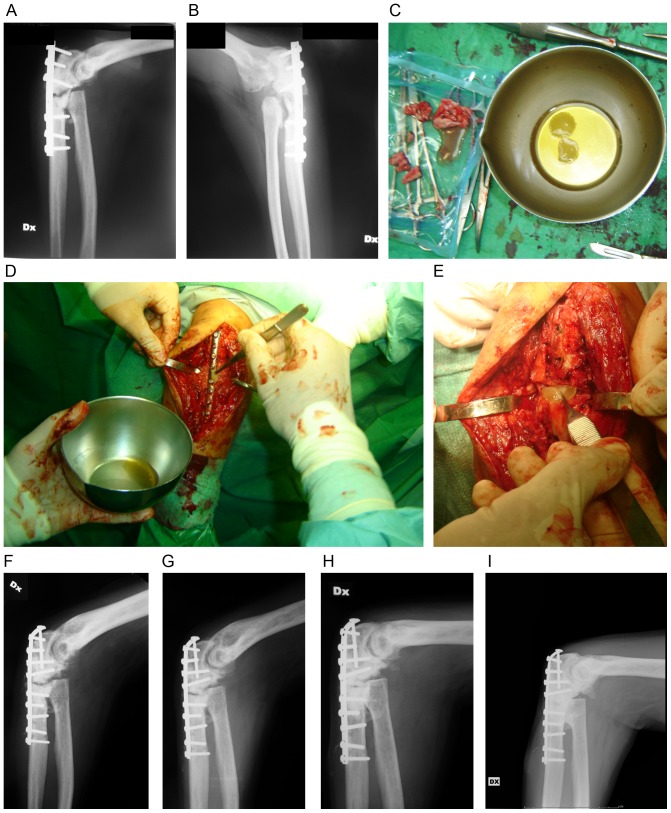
Pre- and post-implant of MSC/fibrin clot constructs in a representative patient (M.51). (A,B) Pre-intervention images showing pseudarthrosis and plate detachment. (C) MSC/fibrin clot constructs and bone chips on the operating table. (D,E) Implant of MSC/fibrin clot constructs during surgical procedure. Radiographic follow-ups at: (F) 2 months; (G) 6 months; (H) 9 months, showing occurred healing and (I) 5 years, showing bone strengthening and absence of side effects.
